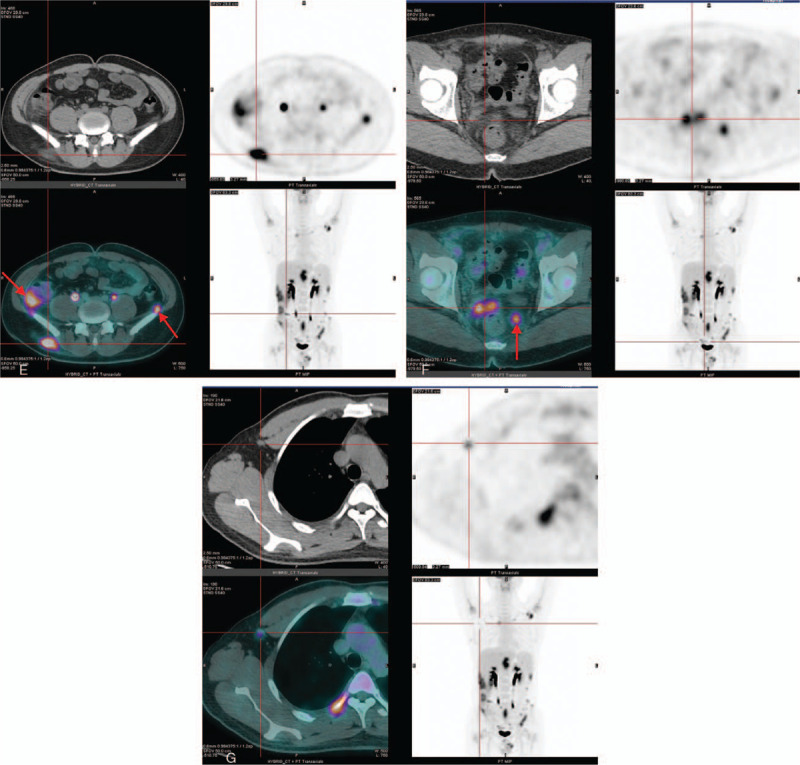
A, The images of fluorine-18 fluorodeoxyglucose positron emission tomography/computed tomography showed a hyper-density plaque with remarkably increased uptake of FDG in the left axilla (SUVmax=7.232). B, a hyper-density FDG-avid lesion within the fat space among the muscles of the left upper chest wall was found. C, the images demonstrated lesion in the right extra-pleural fat with increased density and FDG uptake (SUVmax= 4.506). D, the images showed multiple lesions in the fatty capsule of right kidney and bilateral extra-peritoneal fat, with increased density and FDG uptake. E, the images showed infiltrative lesions within subcutaneous fat tissue in the right hip and colon mesentery (indicated by the arrows). F, FDG-PET images showed hypermetabolism in the areas of mesorectum with slight increase in density. G, a slightly enlarged lymph node presented with abnormal F-18 FDG uptake in the right axilla (SUVmax= 1.944, 9 × 7 mm).
